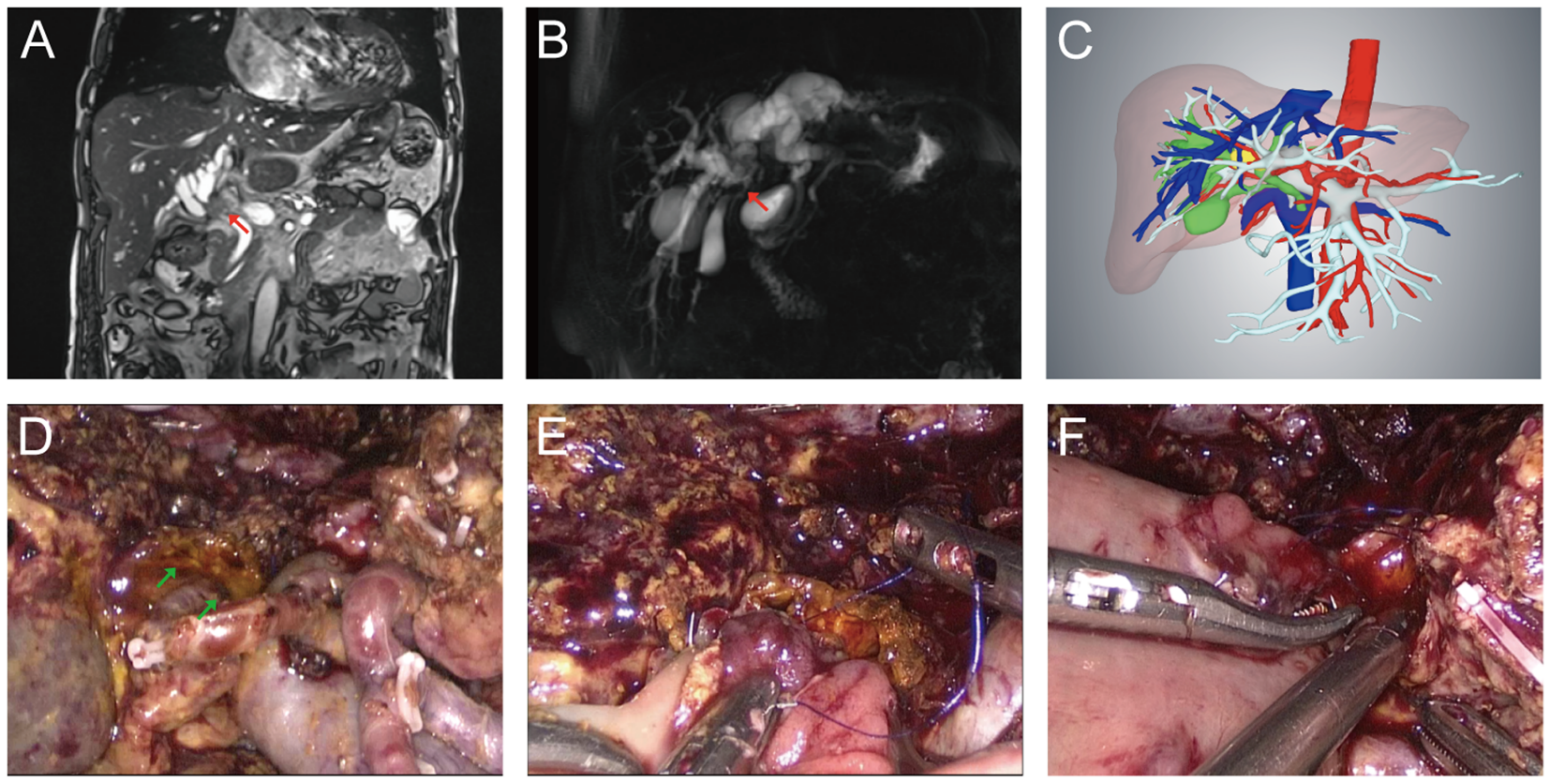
Typical Bismuth type IV HC patient who received laparoscopic radical resection. (A) Preoperative MRI image. The red arrow indicated where the tumor of bile duct was located. (B) Preoperative MRCP image. The red arrow indicated where the tumor was located. (C) Preoperative 3-D reconstruction model of the Bismuth type IV HC patient. The yellow area indicated by the red arrow was the tumor. (D) The hepatic hilum after central hepatectomy and extended lymphadenectomy. Skeletonized portal vein and hepatic artery were visible and the green arrow indicated the bile duct opening after central hepatectomy. (E) Biliary-intestinal anastomosis of the right hepatic duct section. (F) Biliary-intestinal anastomosis of the left hepatic duct section.
